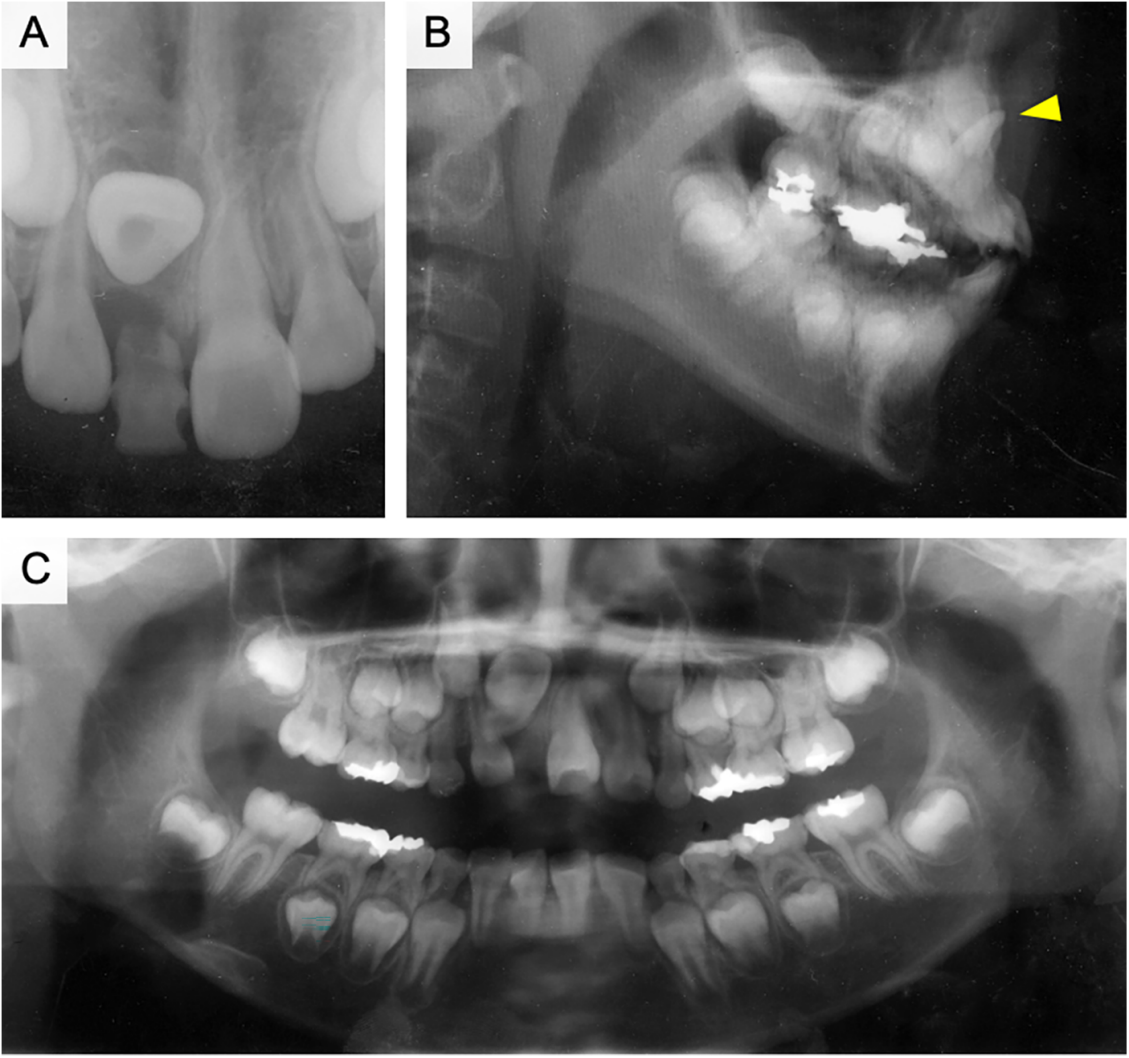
Pre-treatment x-ray photograph of case 1. (A) Dental x-ray photograph taken at the first consultation. (B) x-ray image of the sagittal plane. The red arrowhead is an inversely impacted maxillary central incisor. (C) Panoramic radiograph taken after the extraction of the upper right primary central incisor.
